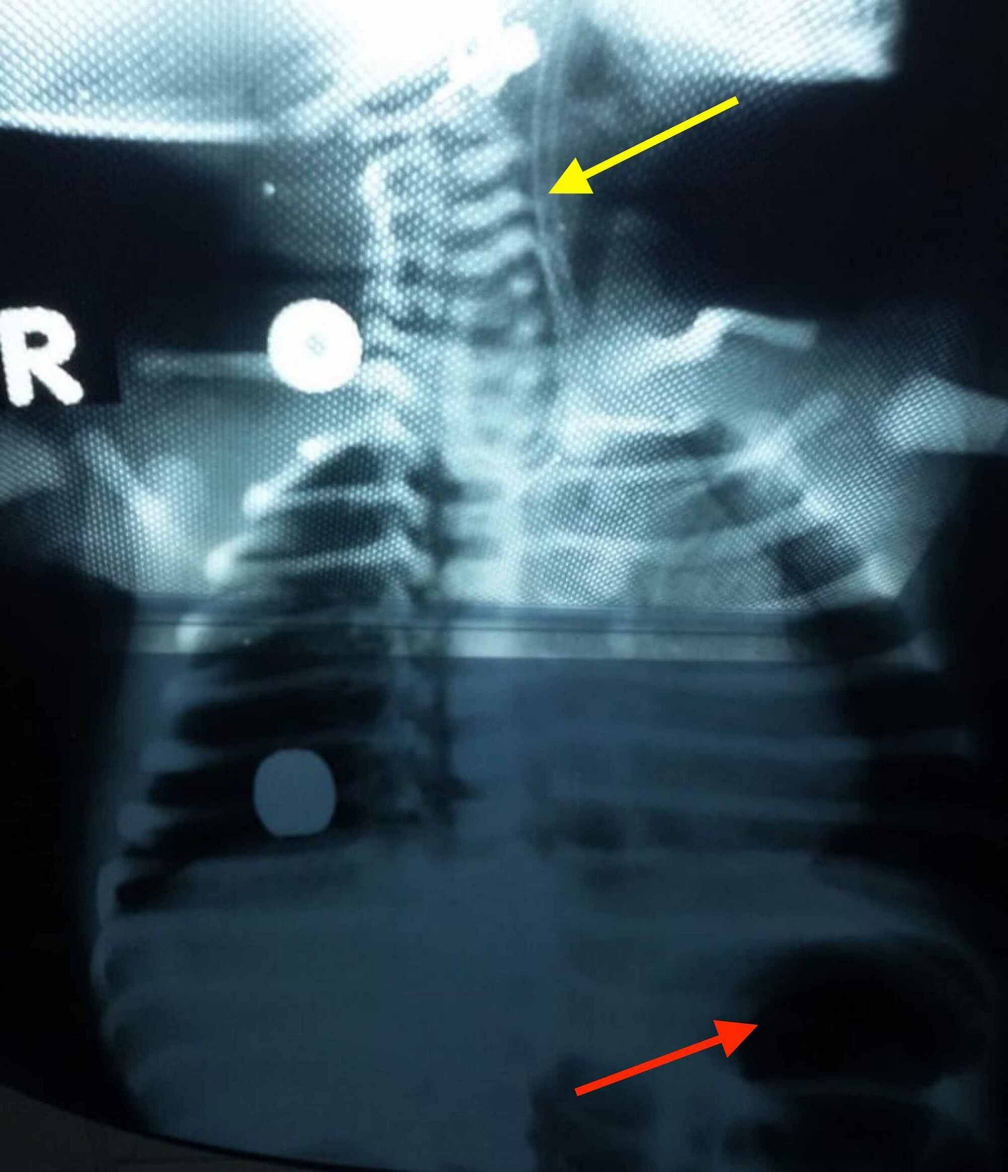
A preoperative plain radiograph demonstrating coiling of the NG tube in the upper pouch of the esophagus (yellow arrow) and gas in the stomach (red arrow), indicating a tracheoesophageal fistula. NG: nasogastric
